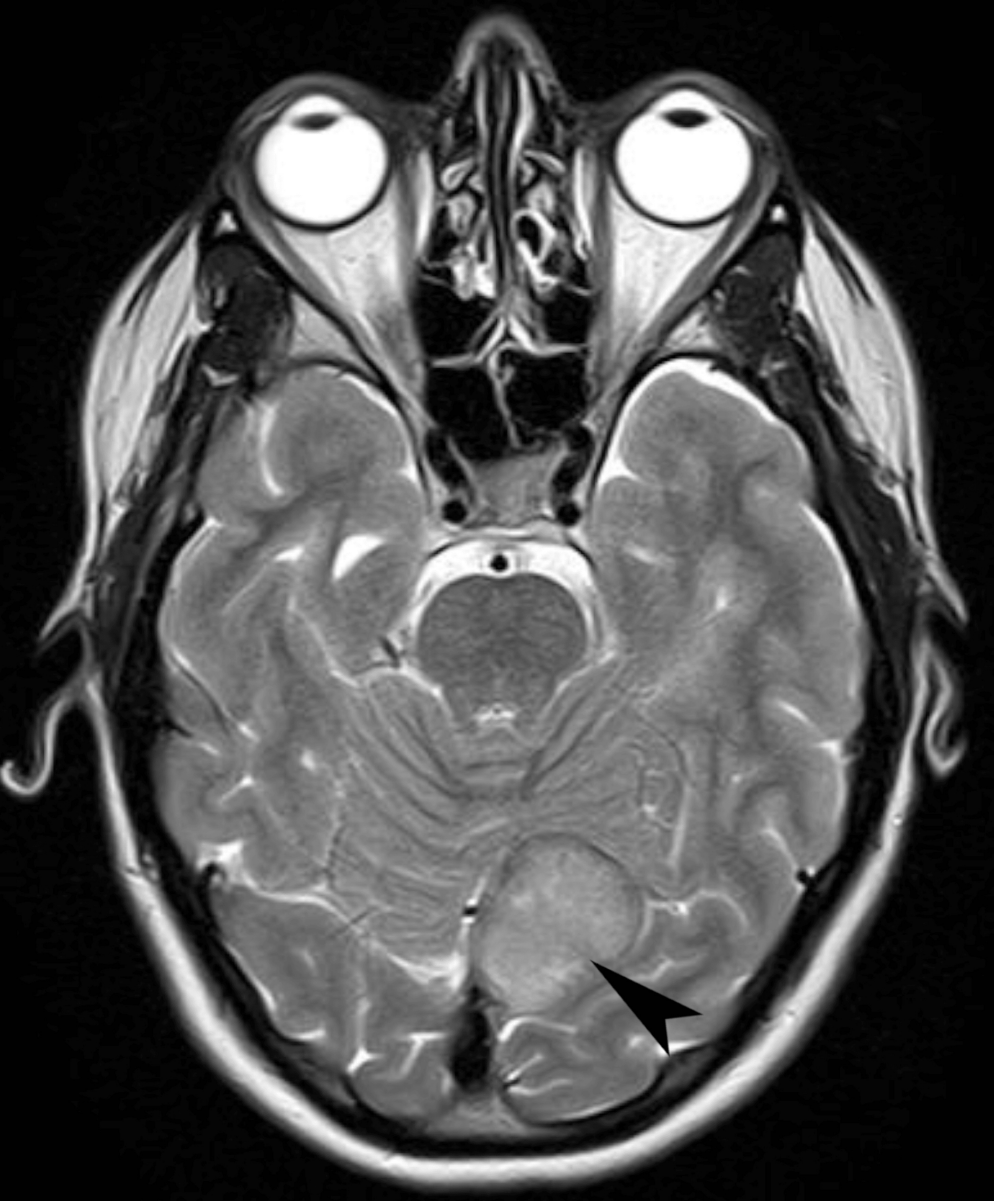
Magnetic resonance imaging of the brain. This axial T2-weighted fast spin echo image further demonstrates the mass (black arrowhead), which is exerting mass effect on the vermis and left cerebellar hemisphere.
